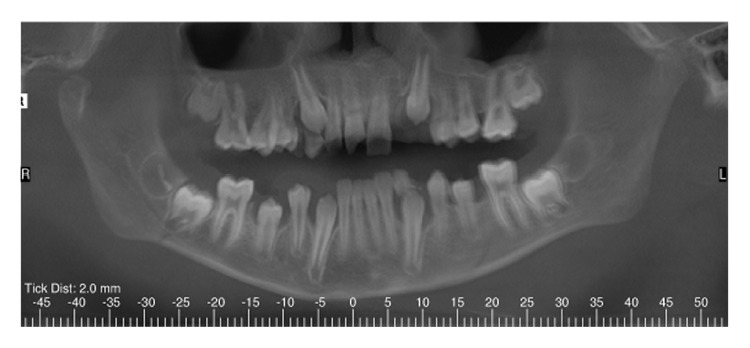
Initial panoramic radiography showing mandibular body fractures, avulsed maxillary left lateral incisor, and maxillary right central incisor with horizontal root fracture.
